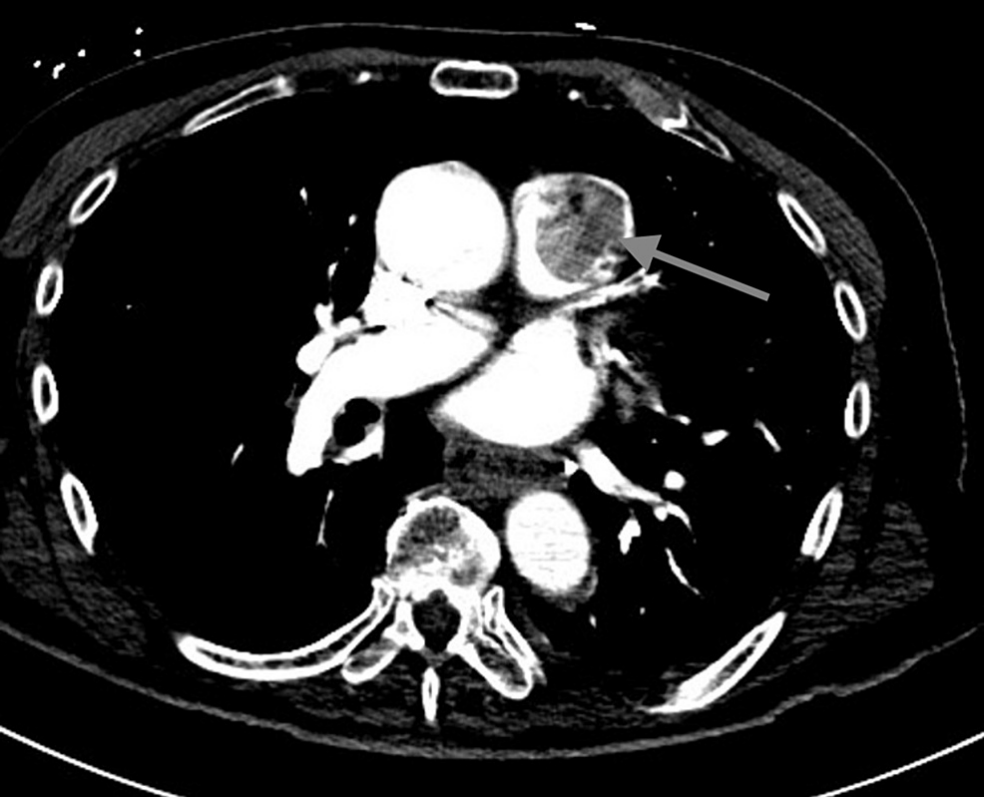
Transverse view CTA of the chest revealed a filling defect in the main pulmonary artery just above the pulmonary valve. CTA, computed tomography angiography
